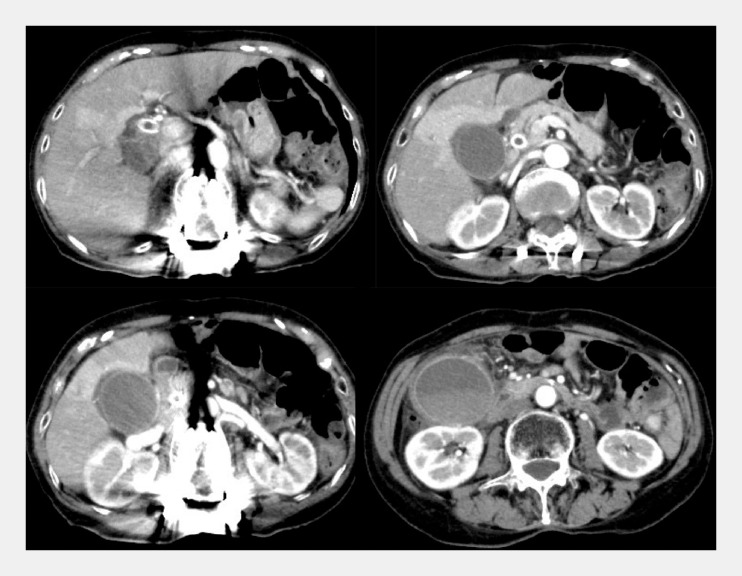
Contrast-enhanced computed tomography performed at the onset of cholecystitis revealed gallbladder swelling. A covered metal stent was placed in the common bile duct.
